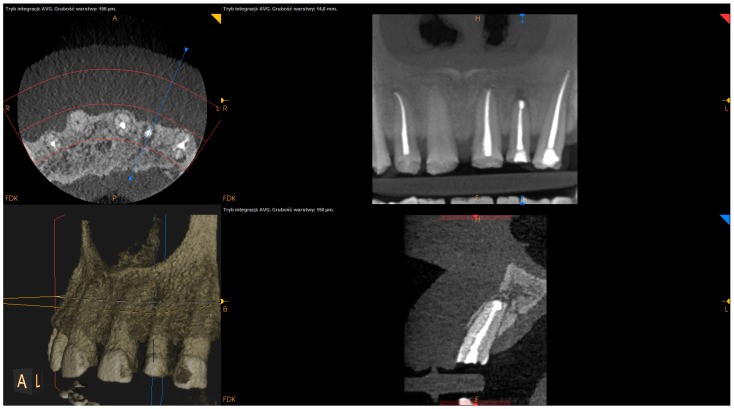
CBCT image (CS 8100 3D Carestream Dental^ⓡ^) example of incomplete healing based on PENN 3D criteria. Non-English annotations present software technicalities (AVG integration mode and layer thickness).
